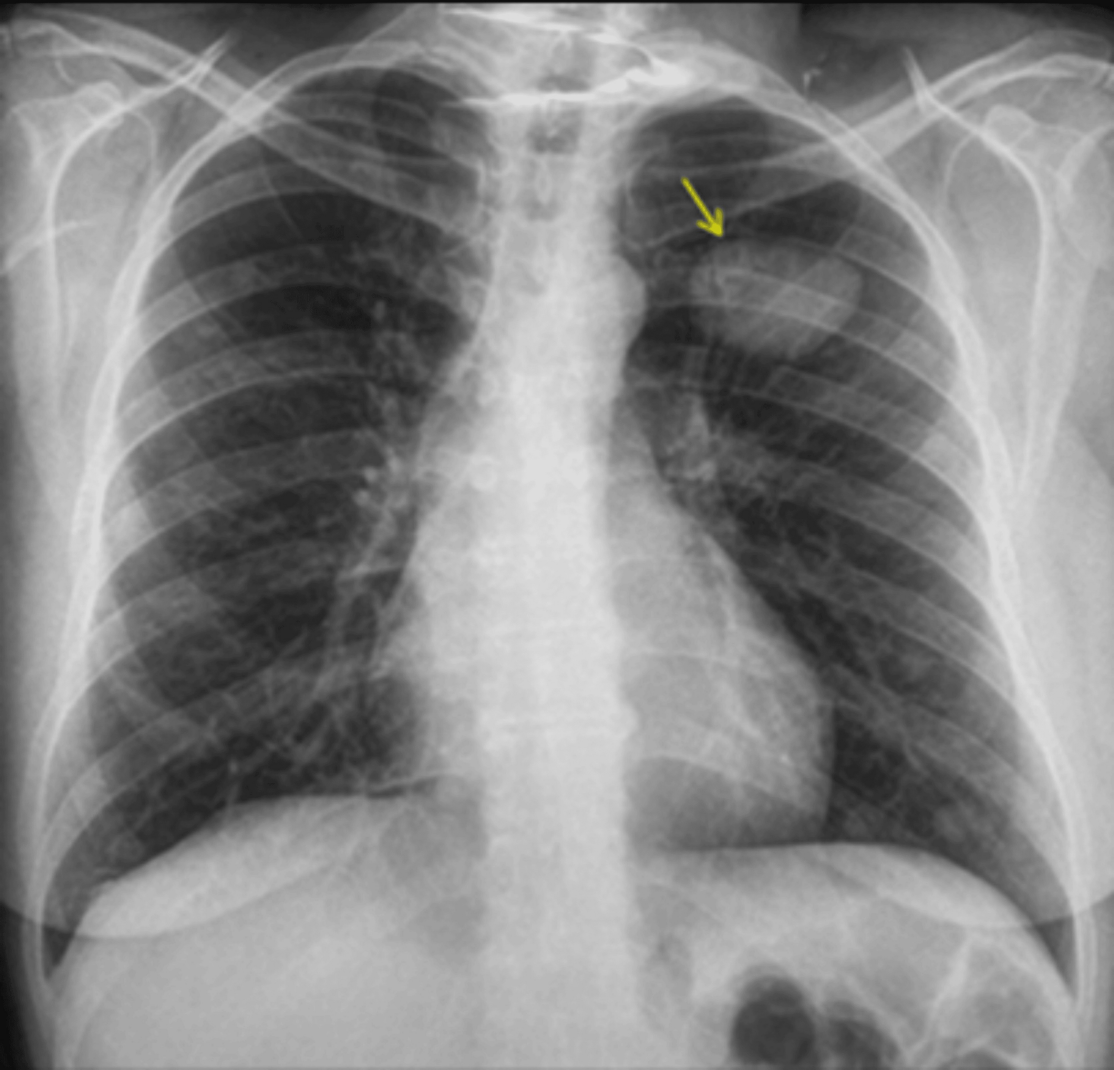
Frontal chest radiograph demonstrates bilateral lung nodules and masses. The largest lesion was noted in the left upper lobe, six years ago.
